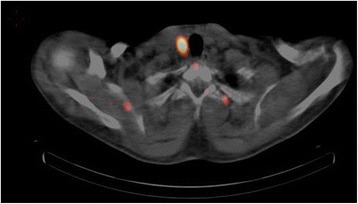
Axial fused cervical single-photon emission computed tomography/computed tomography image showing pathological right parathyroid tissue
